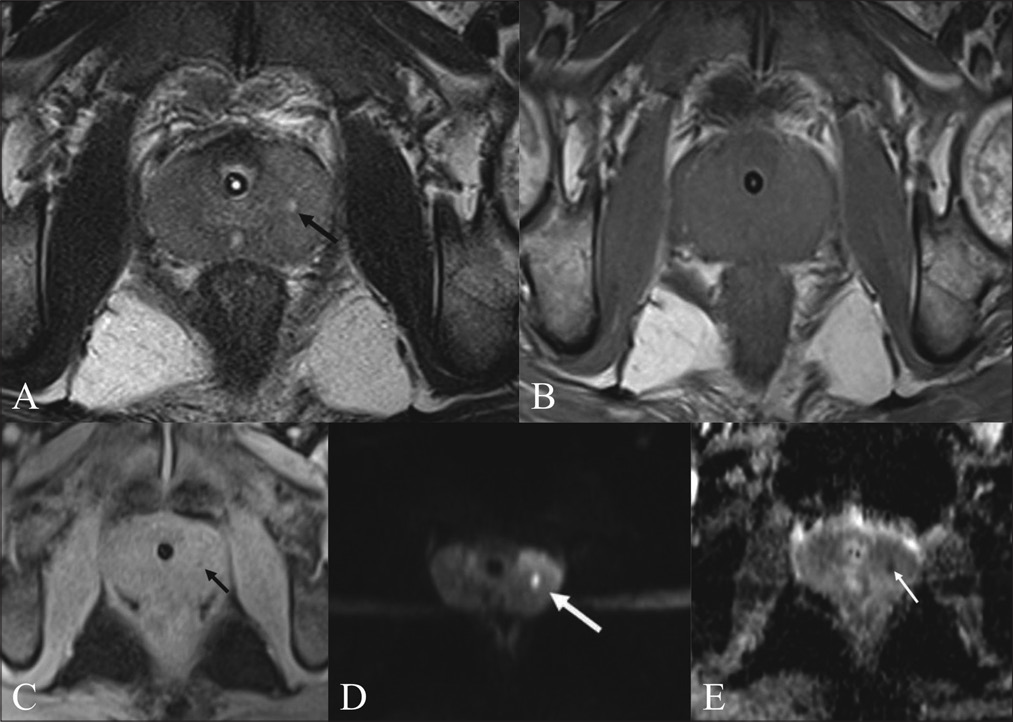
Prostatic abscess – case 1. Axial T2W image (A) of the prostate shows a focus of hyperintense signal (arrow) in the left midzone of the peripheral gland. On the axial T1W image (B), the lesion is barely seen. An axial, contrast-enhanced, T1W image (C) shows that the lesion (arrow) has peripheral enhancement and central non enhancement. DWI (b value=800) (D) shows high signal in the lesion (arrow) due to restriction of diffusion. The corresponding ADC map (E) shows low signal (arrow)
